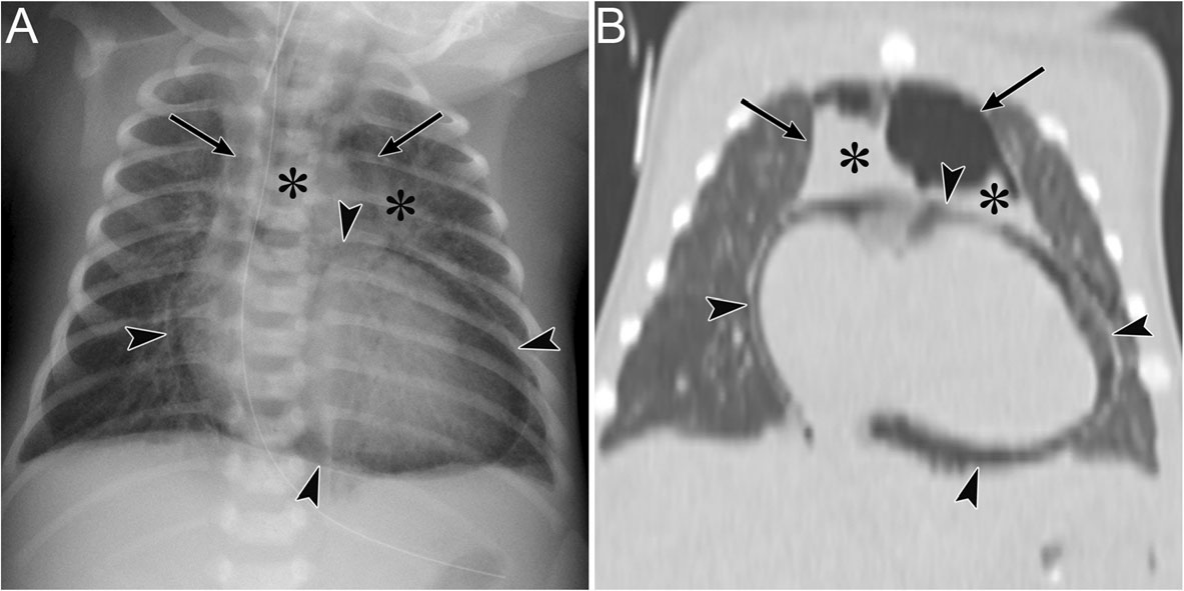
Pneumomediastinum and pneumopericardium in a 3-day-old girl with pulmonary atresia. Pneumomediastinum is caused by the obstruction and check valve effect of the tracheal tube with pulmonary secretions. a A supine radiograph shows the “spinnaker sail” sign with thymus elevation (asterisks) by pneumomediastinum (arrows). Pneumopericardium (arrowheads) is also visible. b A coronal CT image reveals pneumomediastinum (arrows) surrounding the thymus (asterisks) and pneumopericardium (arrowheads)
